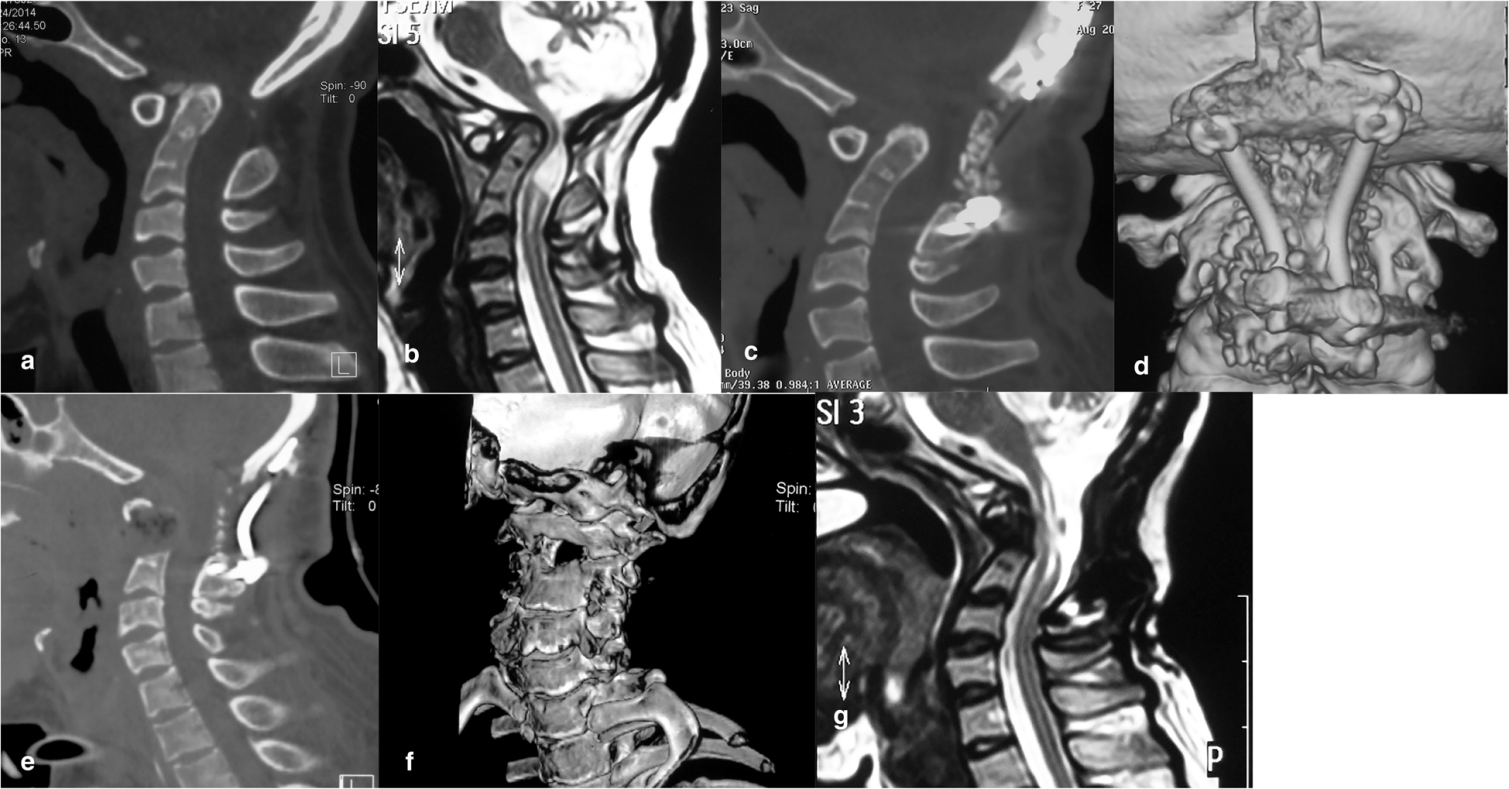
a Sagittal CT image and b sagittal MRI before the first posterior operation, suggesting that the patient had BI and AAD. The spinal cord was compressed from the ventral aspect. c, d Indicating that after posterior distraction and fixation, AAD reduction was not satisfactory, the occipital foramen is enlarged and bone graft fusion is solid. e, f Three months after the operation, CT images suggesting that the odontoid process was fully resected, part of C2 vertebral body was simultaneously resected, and the foramen magnum was further enlarged. g Sagittal MRI revealing decompression of the spinal cord
